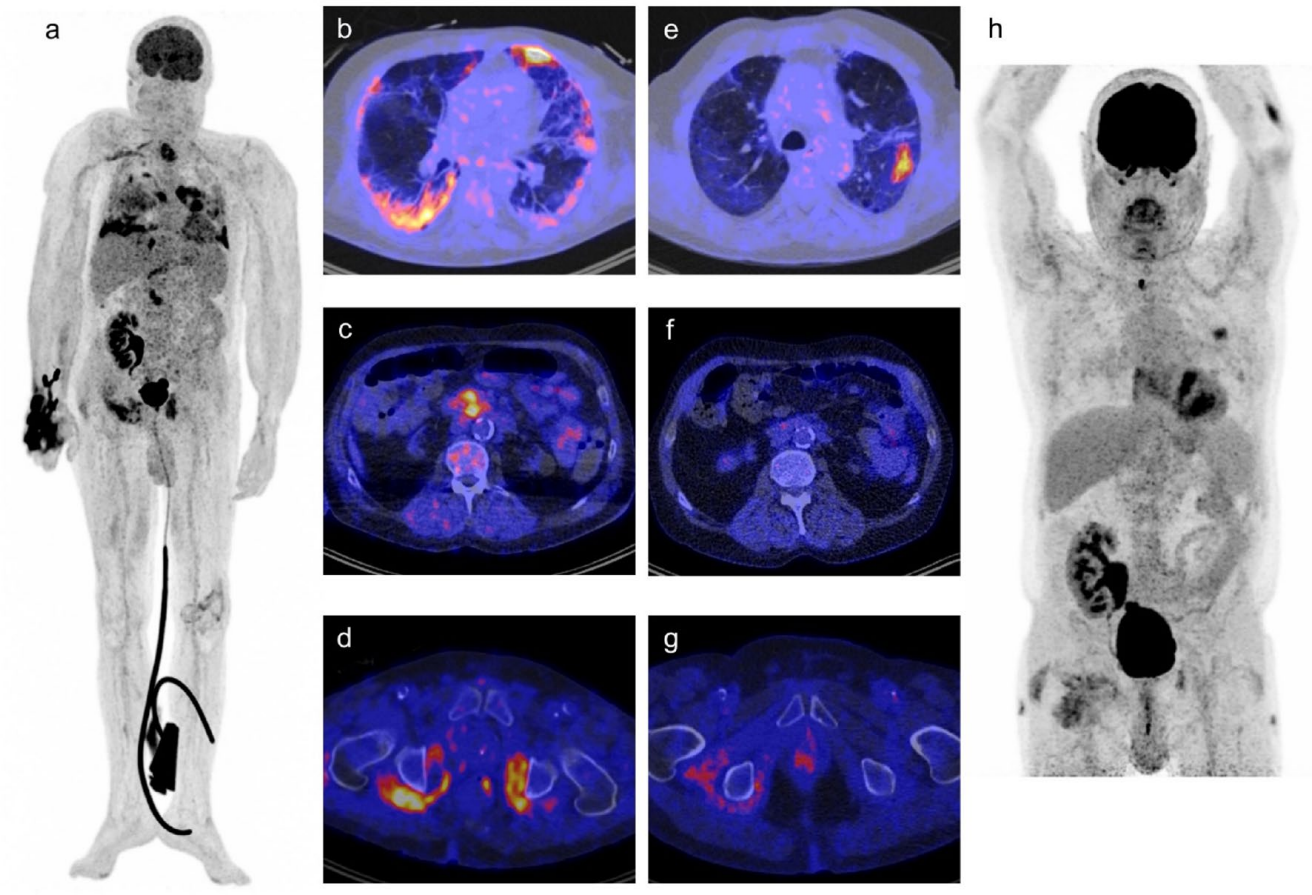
[^18^F]FDG-PET/CT scan of patient 1. This concerns a 65-year-old man who was admitted to the ICU 2 weeks after experiencing symptoms of COVID-19 at the start of the pandemic. An [^18^F]FDG-PET/CT scan was performed 18 days after intubation due to ongoing high CRP levels and negative PCR results for SARS-CoV-2, with no other identified focus of infection. Although not at the time, retrospectively a pancreatitis seems likely due to absence of uptake at the follow-up [^18^F]FDG-PET/CT and high amylase at the time of ICU admission. The [^18^F]FDG-PET/CT did not lead to therapy changes and the patient recovered slowly. [^18^F]FDG-PET/CT revealed high uptake in the muscles in the hip region, which was finally diagnosed as heterotopic ossification. Initial scan: a Maximum intensity projection. Physiological uptake in the right-sided donor kidney, liver, bladder and brain. Percutaneous tracer injection in the right hand. Uptake below the cricoid due to a tracheostomy. Some muscle uptake in the shoulders. B Transaxial pulmonary fusion PET/CT image with clear [^18^F]FDG uptake in the peripheral areas of the lung. CT in lung setting. C Transaxial abdominal fusion PET/CT with [^18^F]FDG uptake in the pancreatic head. CT in soft tissue setting. D Transaxial fusion PET/CT image of the hip region with [^18^F]FDG uptake in the dorsal muscles. CT in bone setting. Follow-up scan: E Transaxial pulmonary fusion PET/CT with only one active lesion left. CT in lung setting. F Transaxial abdominal fusion PET/CT, [^18^F]FDG uptake in the pancreas disappeared. CT in soft tissue setting. G Transaxial fusion PET/CT of the hip with decreased [^18^F]FDG uptake in the dorsal muscles. On CT, some linear calcifications are visible in the right dorsal hip muscles. CT in bone setting. H Maximum intensity projection of follow-up scan. Physiological uptake in the donor kidney, liver, and spleen. Tracheostomy uptake decreased. *SUV-bw threshold of 5.00; α* = *50%*
